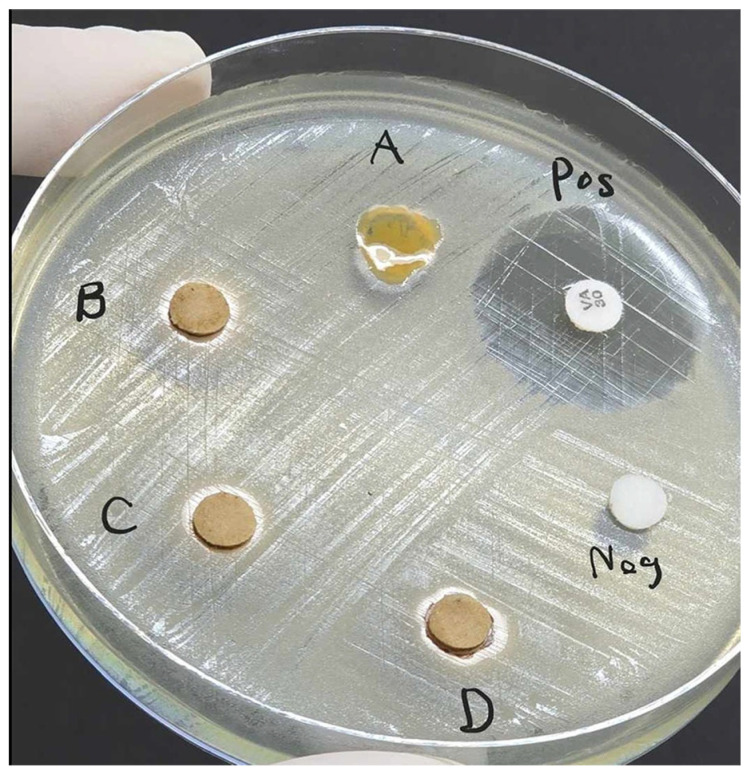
Inhibition of *Streptococcus mutans* by tray-dried cocoa pod husk extracts determined using the disc diffusion method. The extracts were obtained using ethanol (solid-to-solvent ratio of 1:7) and aqueous ethanol (1:3 *v*/*v*) at solid-to-solvent ratios of 1:5, 1:7, and 1:9 (*w*/*v*), followed by probe ultrasound-assisted extraction at an amplitude of 50% for 30 min. A: ethanol extract at a solid-to-solvent ratio of 1:7; B: aqueous ethanol extract (1:3, *v*/*v*) at a solid-to-solvent ratio of 1:9; C: aqueous ethanol extract (1:3, *v*/*v*) at a solid-to-solvent ratio of 1:7; D: aqueous ethanol extract (1:3, *v*/*v*) at a solid-to-solvent ratio of 1:5. Chlorhexidine was used as the positive control, and the extraction solvent was used as the negative control.
